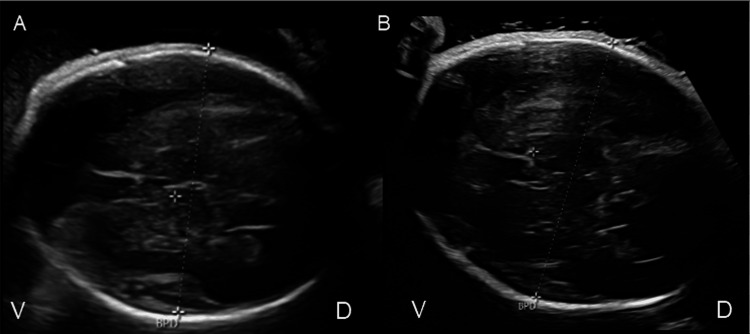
Prenatal ultrasound at (A) 30 and (B) 35 weeks of gestation V: ventral, D: dorsal, BPD: biparietal diameter. Prenatal ultrasound at (A) 30 and (B) 35 weeks of gestation showed no structural abnormality of the fetal brain. Probe marker is directed toward maternal right.
